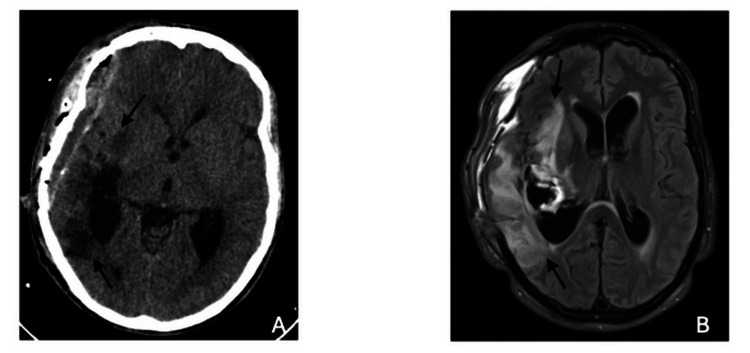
(A) Brain computed tomography scan and (B) magnetic resonance imaging showing ischemic lesions in different evolutionary stages.
